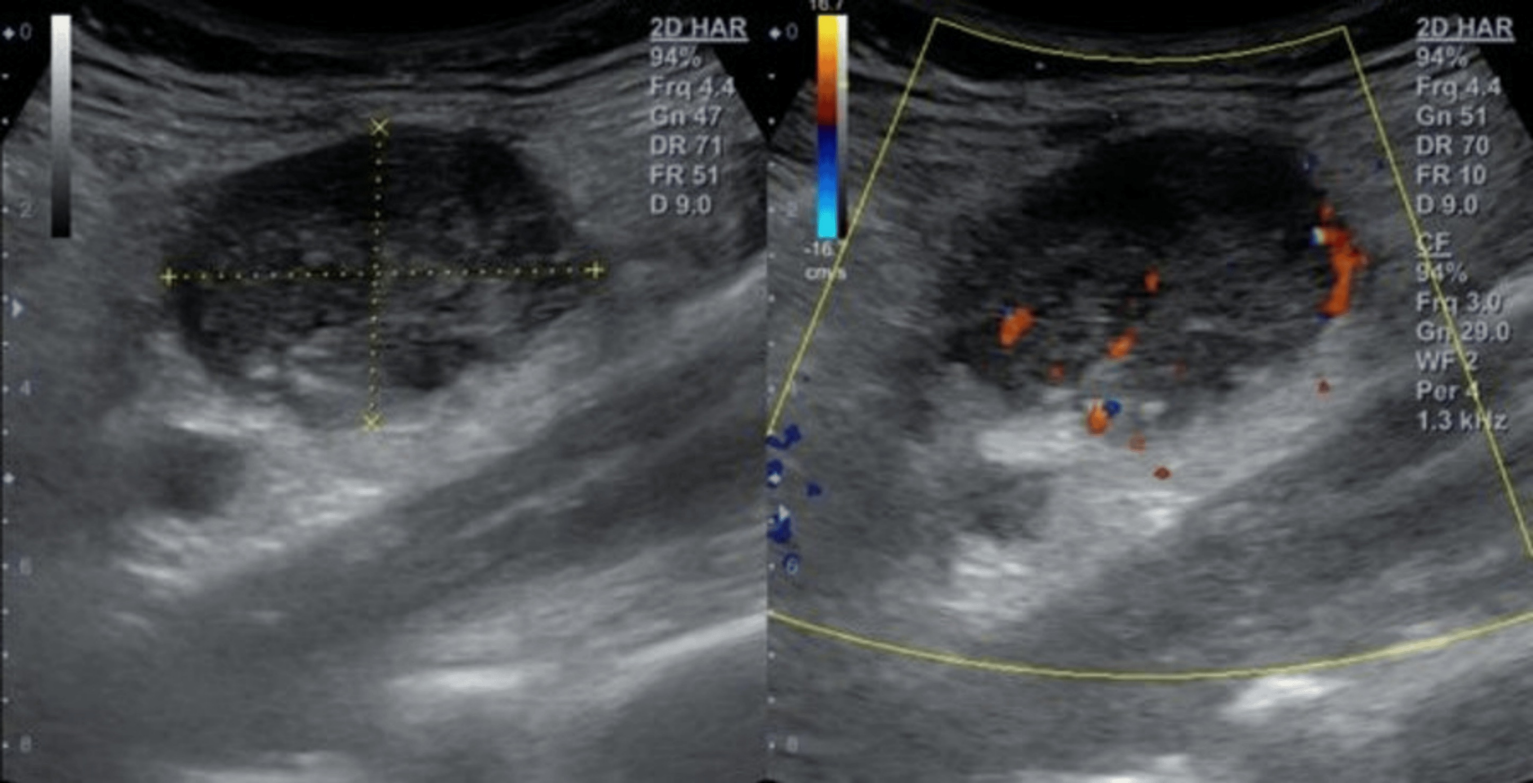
Ultrasonography of the abdomen and pelvis showing a right iliac fossa lump.
